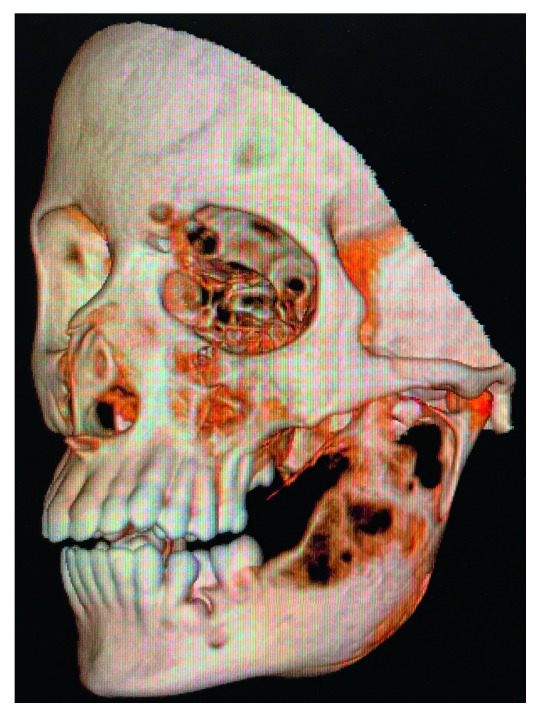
CT scan showing expansile lytic lesion of left ramus of the mandible.
